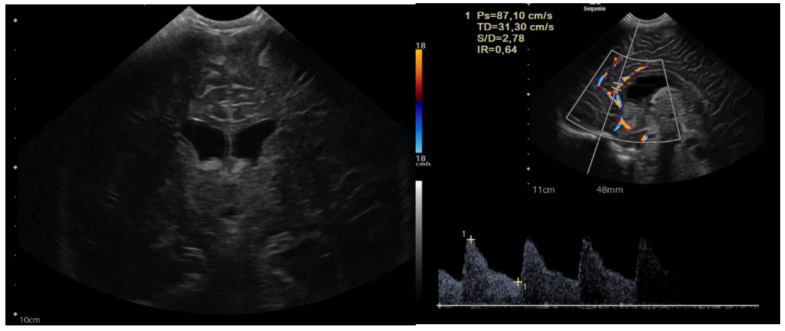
Sagittal and coronal US showing dilated lateral ventricles. Echocolordoppler showed augmented resistance and pulsatility indexes, speaking in favor of augmented intracranial pression.
